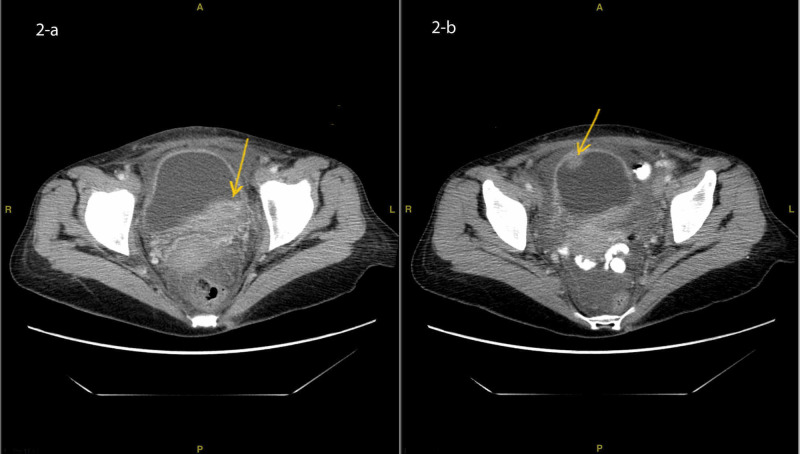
CT scan of the abdomen and pelvis showing the posterior 2.5 x 6.6 cm bladder mass (a) and anterior 1.3 x 2.1 cm bladder mass (b)
